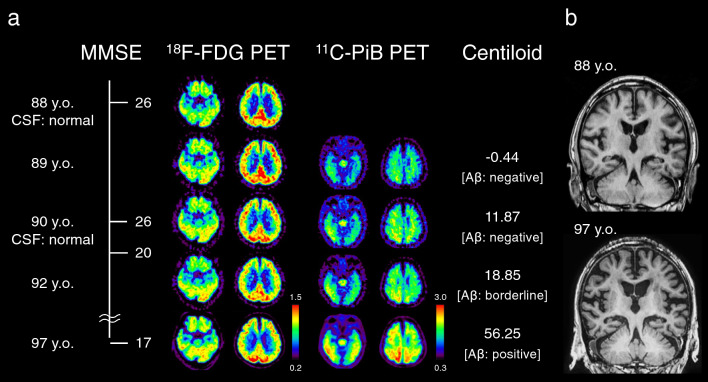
Chronological clinical and positron emission tomography (PET) findings (a). Presenting with mild cognitive impairment, bilaterally reduced radioligand uptake in the medial temporal lobes was observed on [18F]-2-fluoro-2-deoxy-D-glucose (FDG)-PET. However, cerebrospinal fluid (CSF) amyloid β (Aβ), phosphorylated tau, and tau levels were normal, and [11C]-Pittsburgh compound-B (PiB) PET also indicated no significant Aβ deposition. After that, with the progression of cognitive dysfunction, there was a gradual increase in Aβ accumulation in the cerebrum. Centiloid scaling was used to standardize the results of amyloid PET quantitatively. Centiloid scale values range from 0 to 100, with 0 representing definitively Aβ-negative brains of young, healthy controls and 100 representing the average signal observed in patients with mild to moderate dementia due to Alzheimer’s disease. Centiloid scale transition also supports the fact that Aβ accumulation began after the onset of dementia and showed a slow increase. Color bars indicate the standardized uptake value ratio (SUVR). Comparison of T1-weighted MRI images at ages 88 and 97 (b). The overall brain atrophy progressed only modestly despite the 9-year follow-up interval under the dementing condition, while the hippocampal atrophy became more pronounced, extending to the posterior regions. Abbreviations: *MMSE* Mini-Mental State Examination score, *y.o.* years old
